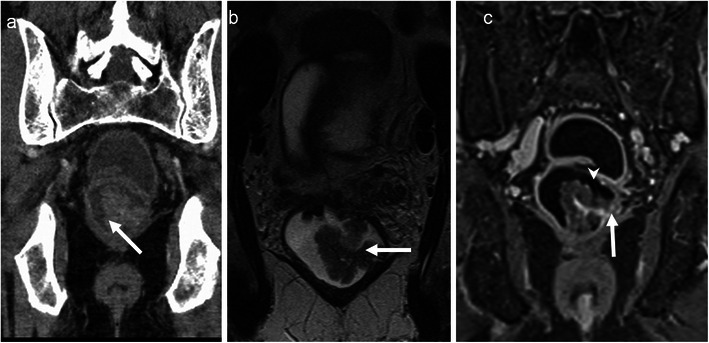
A 65-year-old woman presenting with intermittent diarrhoea, rectal bleeding and difficulty in defecation for 1 month. Laboratory data revealed hypokalaemia. a Coronal contrast CT reveals a lobulated solid mass (*arrow*) projecting into the lumen of the rectum. b Coronal T2-weighted MRI reveals the frond-like morphology of the lesion (*arrow*). c Coronal contrast MRI depicts a central vascular stalk (*arrow*) and the cauliflower or frond-like morphology (*arrowhead*), classical for villous adenoma
